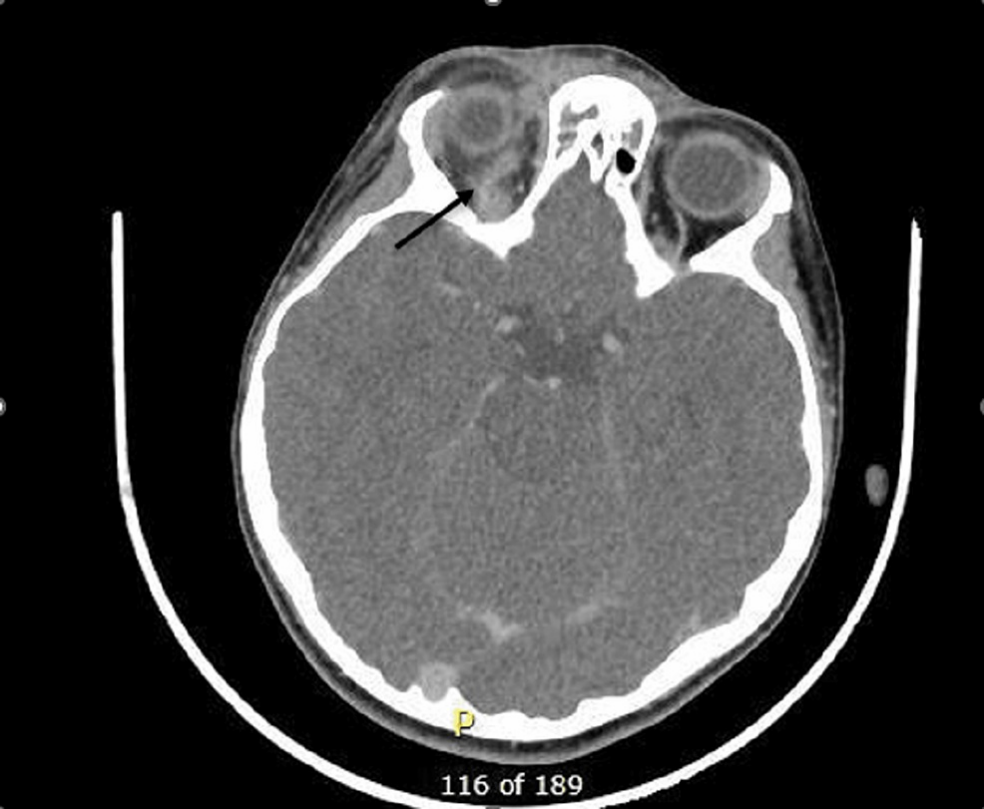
Post-contrast CT (axial view) showing right superior ophthalmic vein thrombosis with vein enlargement in comparison to the left side.
